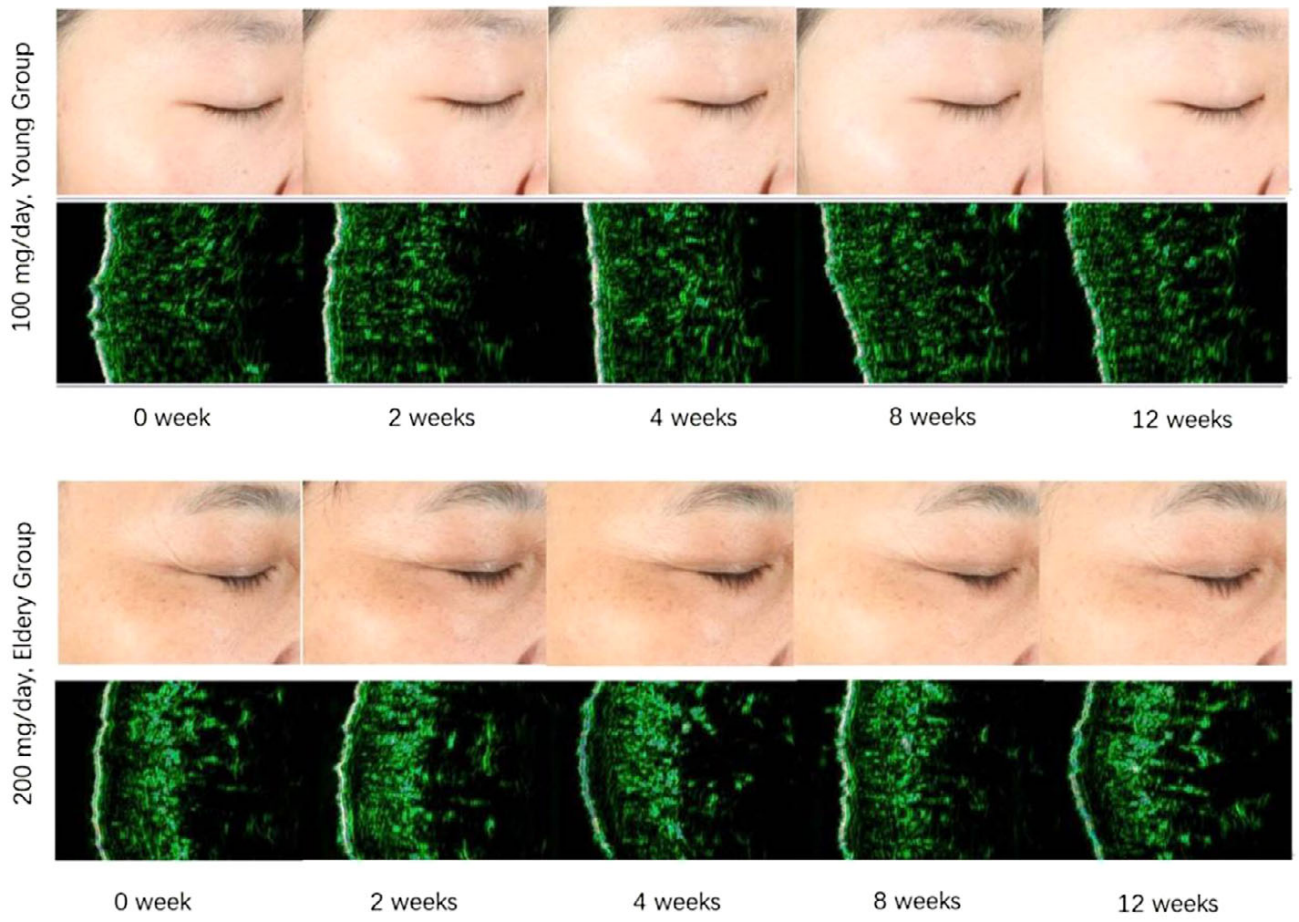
Visia and ultrasound skin diagnostic photos of subjects in the low dose and high dose groups.
